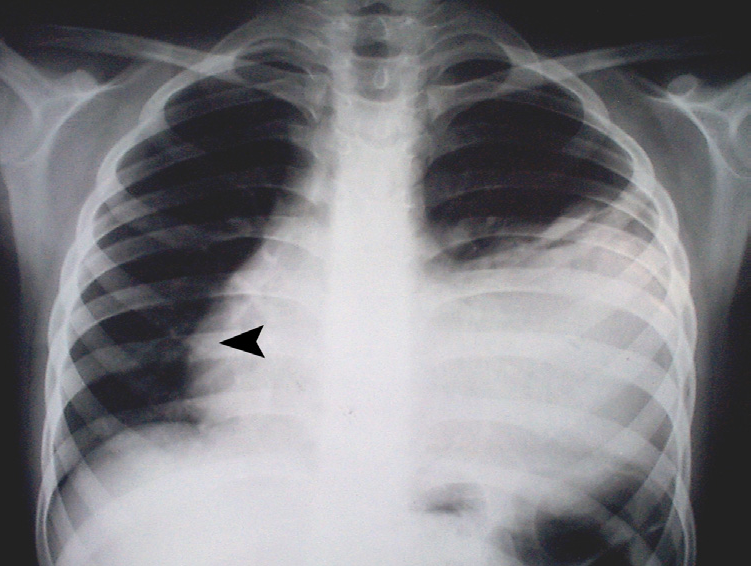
The PA chest X-ray: left-sided pleural effusion and a large mass in anterolateral part of left lung which had overshadowed the left border of the heart. Arrowhead indicates shift of the heart to the right side.
